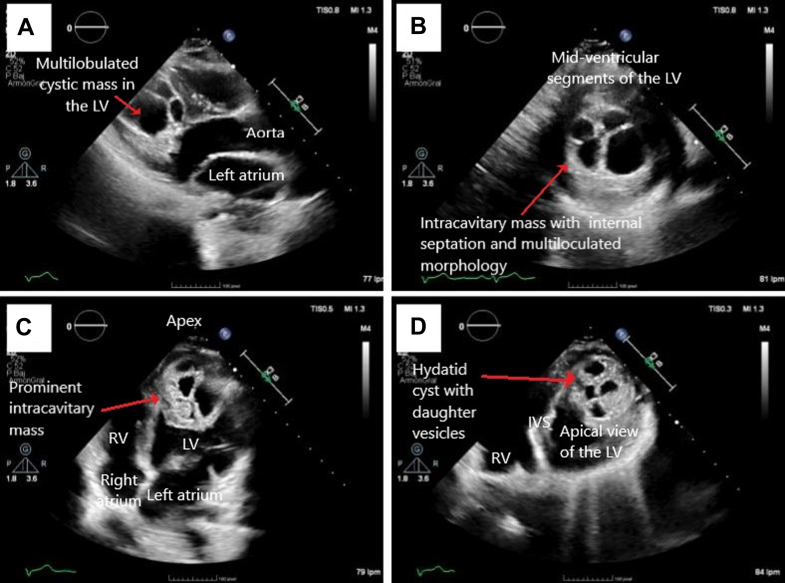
Transthoracic Echocardiographic Images Showing a Large Multiloculated Cystic Intracavitary Mass in the LV, Suggestive of a Hydatid Cyst With Daughter Vesicles (A) Parasternal long-axis view showing a heterogeneous cystic lesion confined to the apical and mid-level regions of the LV. (B) Parasternal short-axis view at the mid-ventricular level, showing internal septation and multiloculated morphology. (C) Apical 4-chamber view with a prominent intracavitary mass extending toward the apex. (D) Modified apical 4-chamber view centered on the apex, clearly delineating the extent of the cystic lesion. IVS = interventricular septum; LV = left ventricle; RV = right ventricle.
